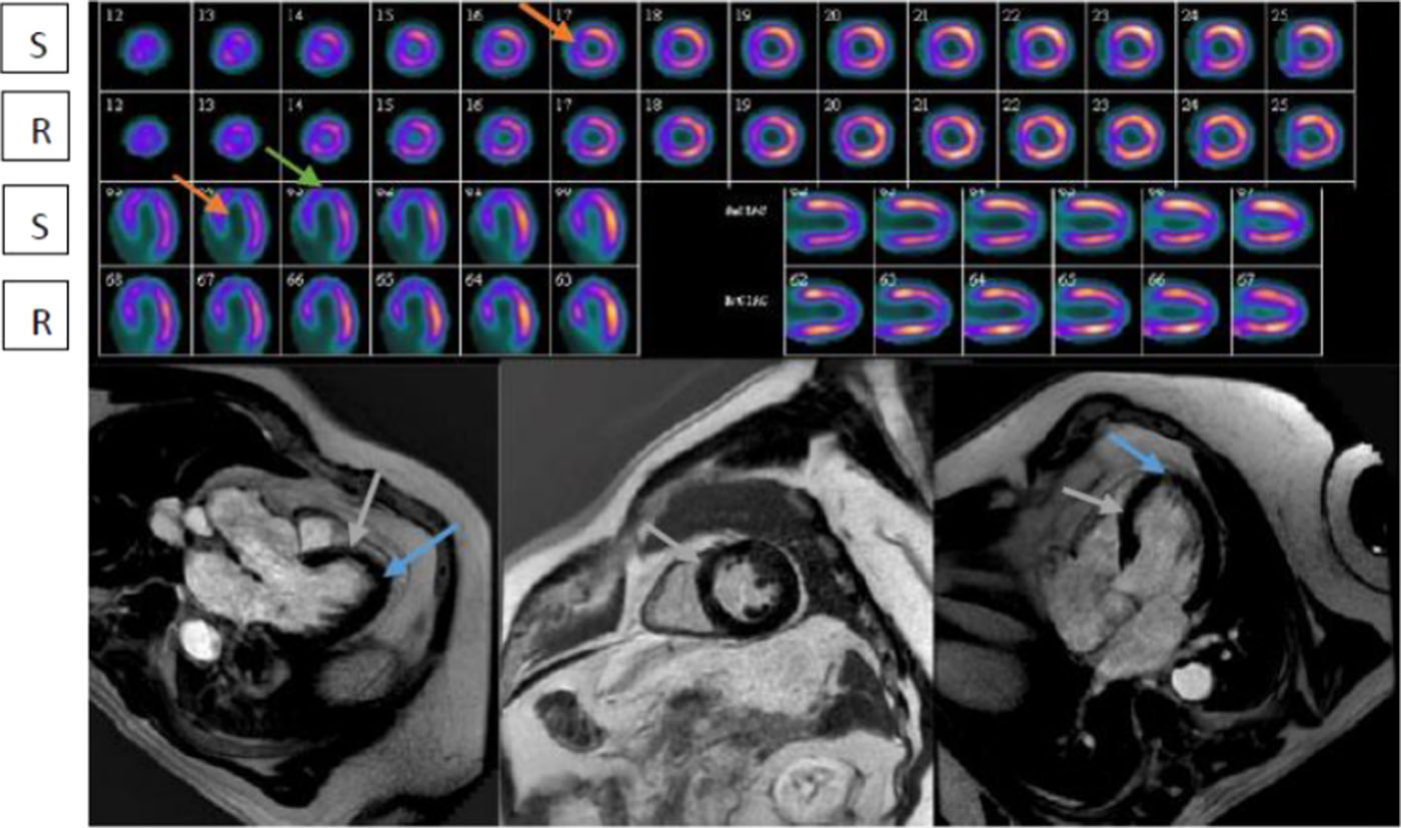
Fixed perfusion defect of the apex and septum on SPECT suggests infarct, however, characterized as VM on CMR with no LGE. LV septum (red arrow) and apex (green arrow) reveal fixed perfusion defect on strss and rest SPECT imaging (A) suggestive of NVM; on CMR (B) septum (gray arrow) and apex (blue arrow) reveal VM (no scar on LGE imaging). CMR, cardiac magentic resonance; LGE, late gadolinium enhancement; NVM, nonviable myocardium; R, rest attenuation corrected; S, stress attenuation corrected; SPECT, Single Photon Emission Computed Tomography; VM, viable myocardium
